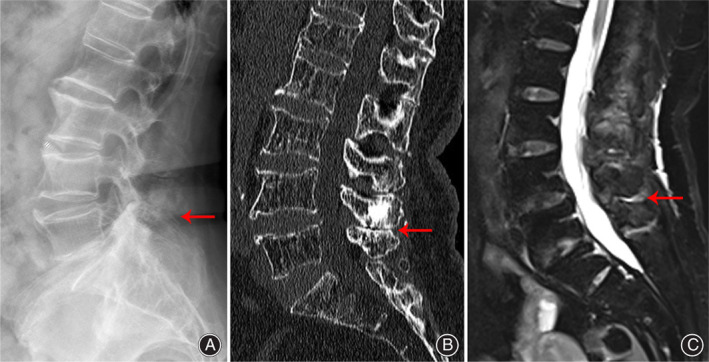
The preoperative imaging data of the patient. (A) X‐ray showed apposition of the dorsal spinous processes. (B) CT showed severe hyperostosis and osteosclerosis on the edges of the L_4_ and L_5_ spinous processes. (C) MRI showed a high signal on fat‐suppressed T2‐weighted images around the L_4_ and L_5_ spinous processes.
